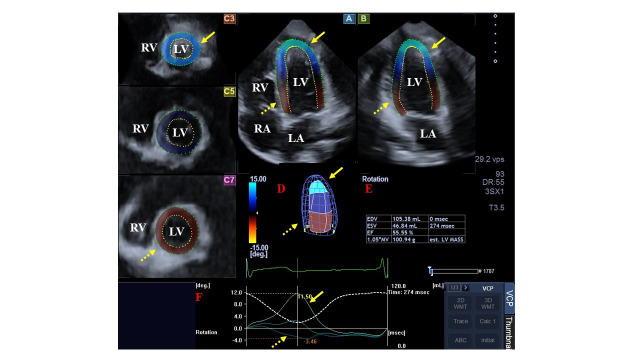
Assessment of the left ventricular rotational mechanics by three-dimensional speckle-tracking echocardiography. Quantification of left ventricular (LV) rotational mechanics via three-dimensional speckle-tracking echocardiography (3DSTE) in a healthy subject presents apical four-chamber (A) and two-chamber (B) long-axis views, along with short-axis views at basal (C3), midventricular (C5), and apical (C7) LV levels. These are shown alongside a 3D LV cast (D) and calculated LV volumes (E), while LV apical (yellow arrow) and basal (dashed yellow arrow) regions with corresponding time-LV rotation curves (coloured curves) are presented with the time–LV volume change curve (dashed white curve) during the heart cycle (F). Abbreviations: ESV, end-systolic volume; EDV, end-diastolic volume; EF, ejection fraction; RV, right ventricle; RA, right atrium; LV, left ventricle; LA, left atrium.
